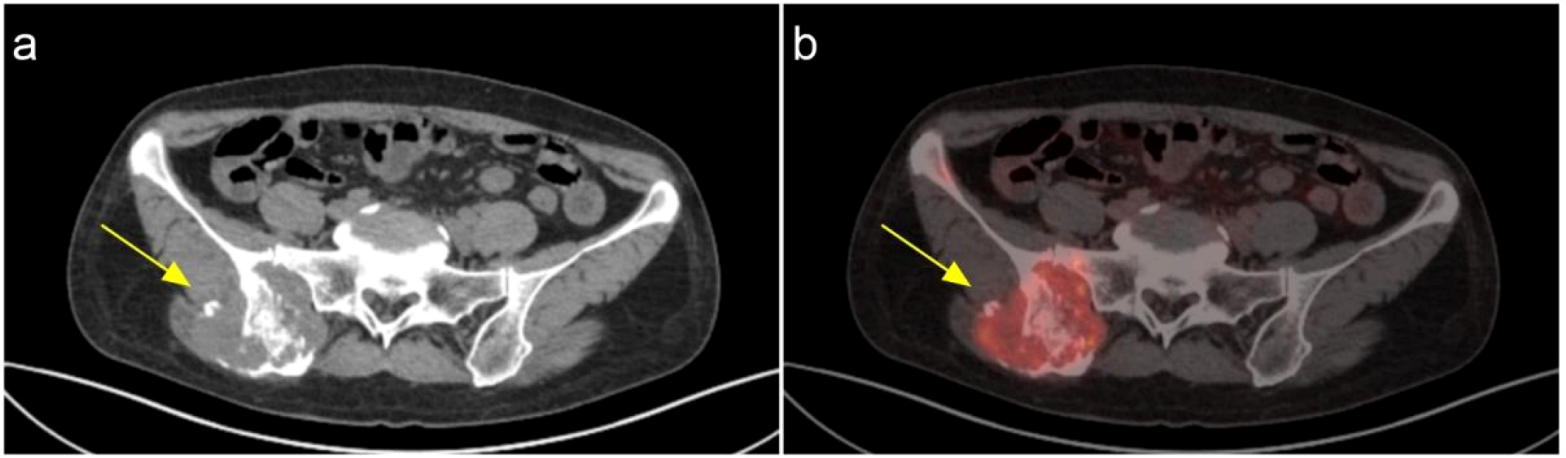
The iliac bone on the right side demonstrates bone destruction accompanied by the formation of a surrounding soft tissue mass, with involvement of the adjacent sacroiliac joint and iliac wing of the sacrum. Increased glucose metabolism is observed, which is consistent with the radiological manifestations of metastatic tumors. (A) CT (B) PET-CT.
